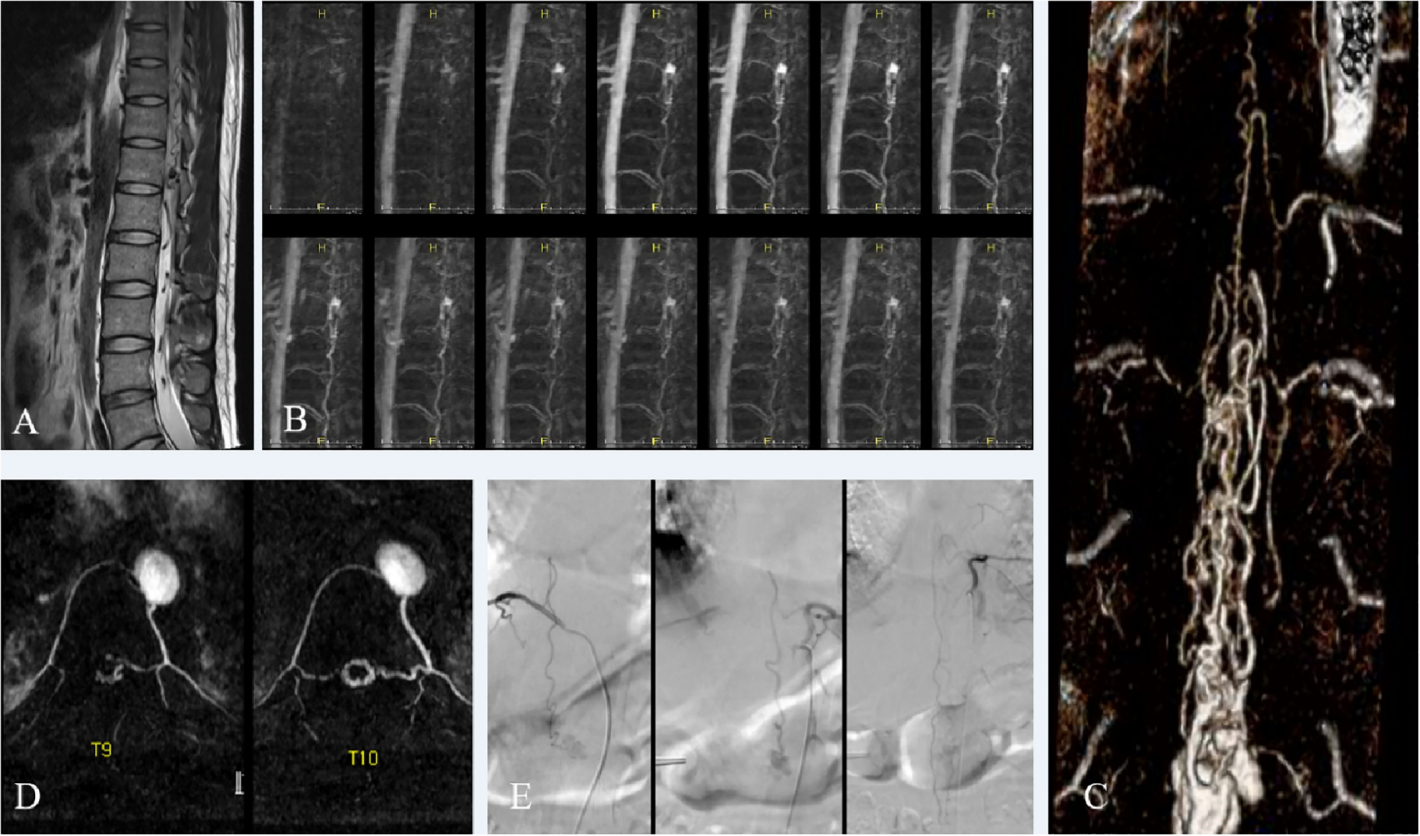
A 31-year-old man with spinal arteriovenous malformation (AVM) (patient 5). Sagittal T2-weighted image (A) revealed tortuous vascular signal voids. Serial maximum intensity projection (MIP) images of low-dose time-resolved contrast-enhanced magnetic resonance angiography (CE-MRA) (B) revealed early visualization of a dilated venous sac. Volume-rendering reconstruction image (C) and segmental MIP images (D) of single-phase high-resolution CE-MRA revealed arterial feeders of the shunt, including the anterior spinal artery arising from the left T9 intercostal artery and posterior spinal arteries from both T9 intercostal arteries. Spinal digital subtraction angiography (E) confirmed spinal AVM with matched level of feeding arteries.
